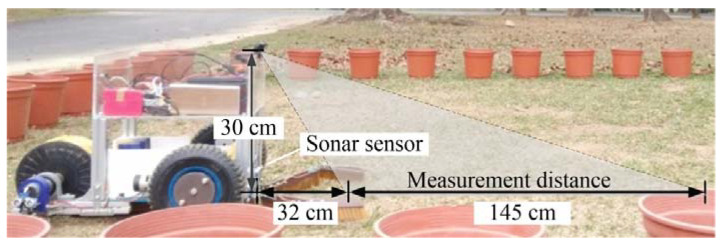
Determination of the visual and ultrasonic detection range for the robot.
